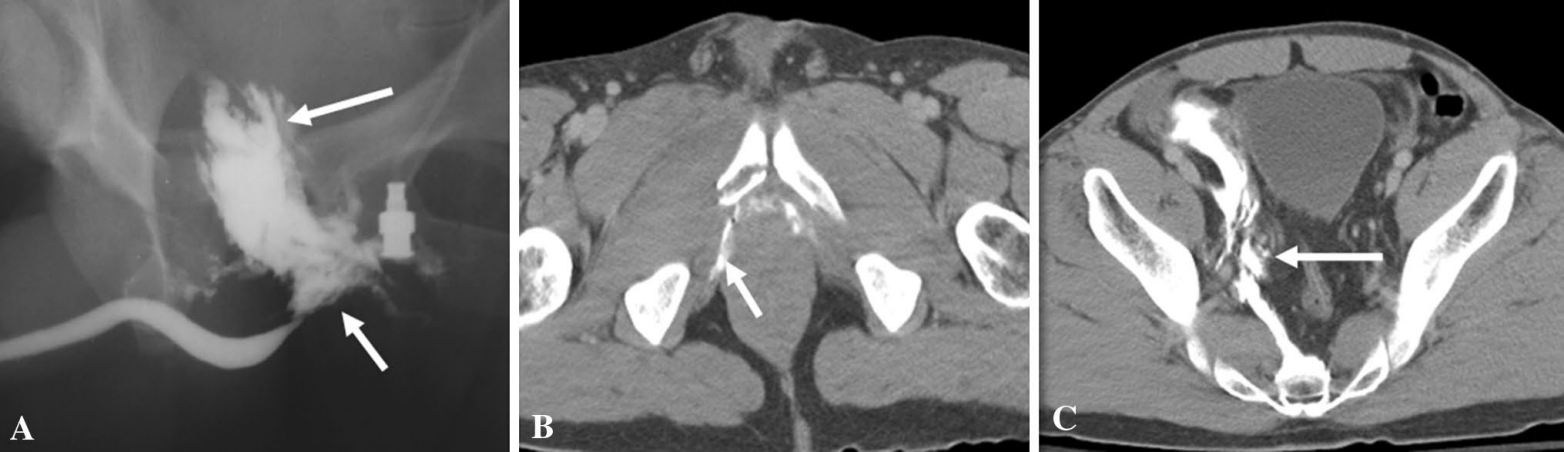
Colapinto–McCallum type III injury. a Retrograde urethrogram shows contrast extravasation from the posterior urethra extending into perineum (short arrow) and pelvis (long arrow). b, c Axial computed tomography of pelvis confirms the perineal (short arrow) and extraperitoneal pelvic extravasation (long arrow)
